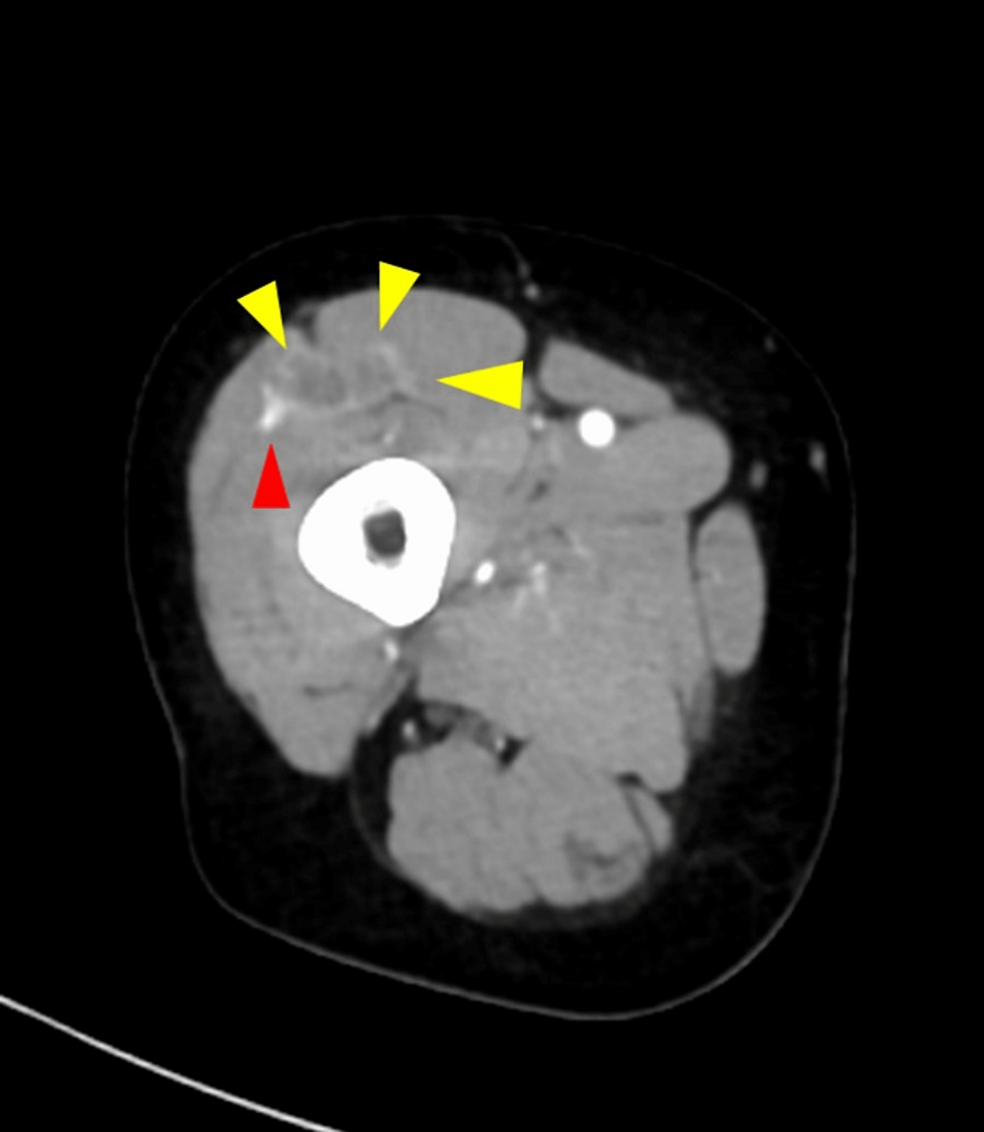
Contrast-enhanced CT of the thigh Metastases (yellow arrow) around the right lateral femoral circumflex artery (red arrow) were observed.
